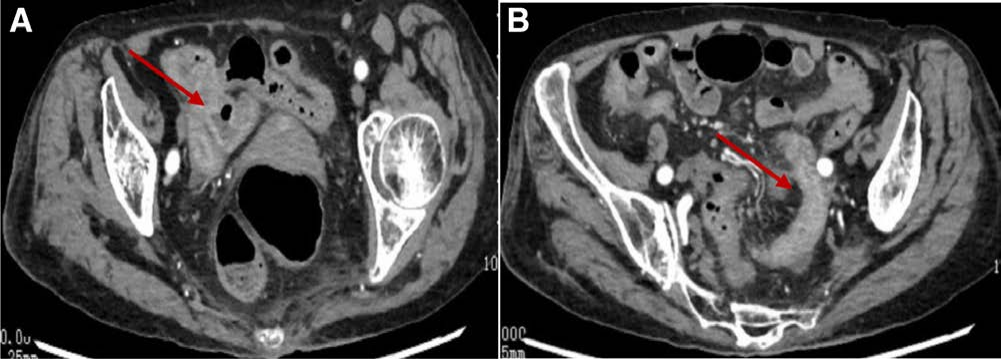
Abdominal enhanced CT shows the partial mildly dilated colon with multiple fluid levels (A) and the edematous and thickened intestinal wall of the ileum (A; arrows). CT = computed tomography.
